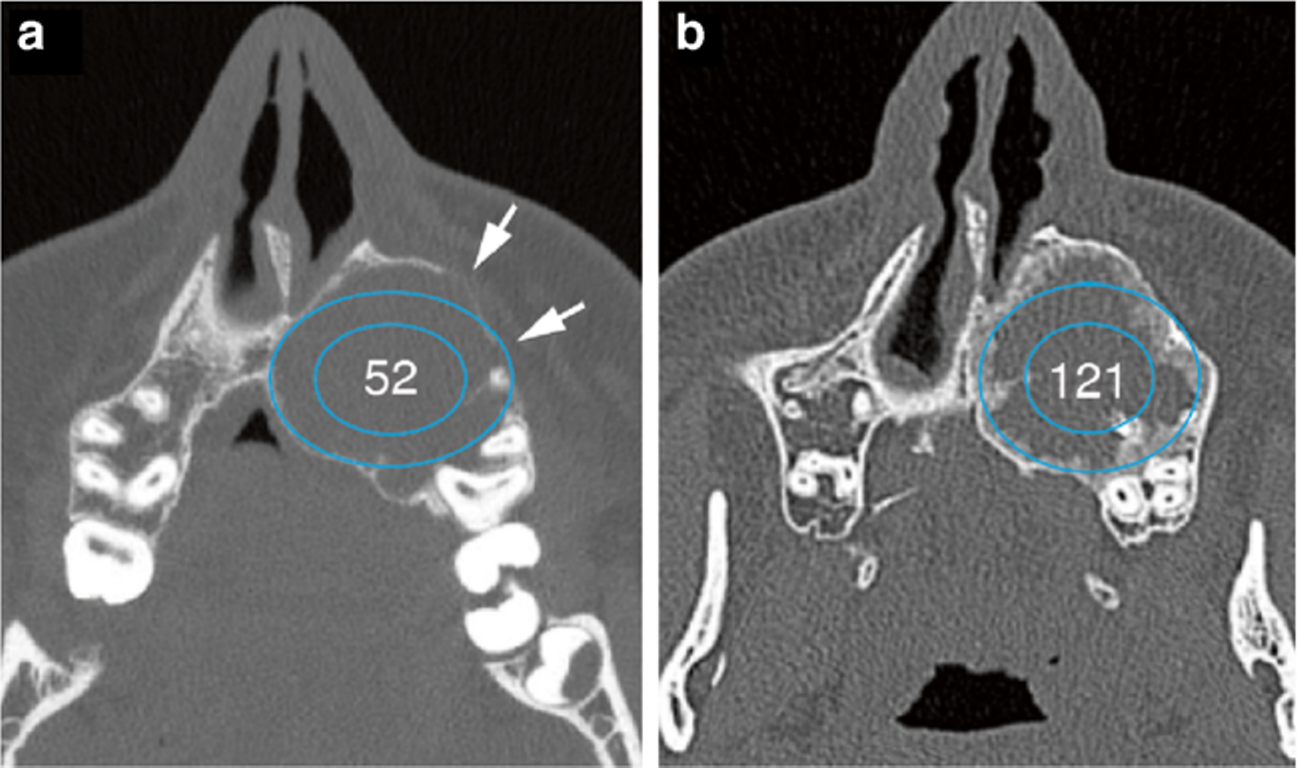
A 15-year-old male presented with swelling of the hard palate, which was first noticed by the dentist. Axial CT of the maxilla at presentation (a) and after 14 weeks on denosumab (b). a CT at presentation shows an expansile lytic GCTB between the roots of the teeth. The cortex was thinned with focal areas of cortical destruction (arrows). No ground glass or chondroid matrix was present in the lesion. The density of the tumour was 52 HU. b Follow-up CT after 14 weeks on treatment shows peripheral sclerosis surrounding the lesion with no cortical defects. The density of the tumour increased to 121 HU. Note that the angulation of the CTs varies slightly
